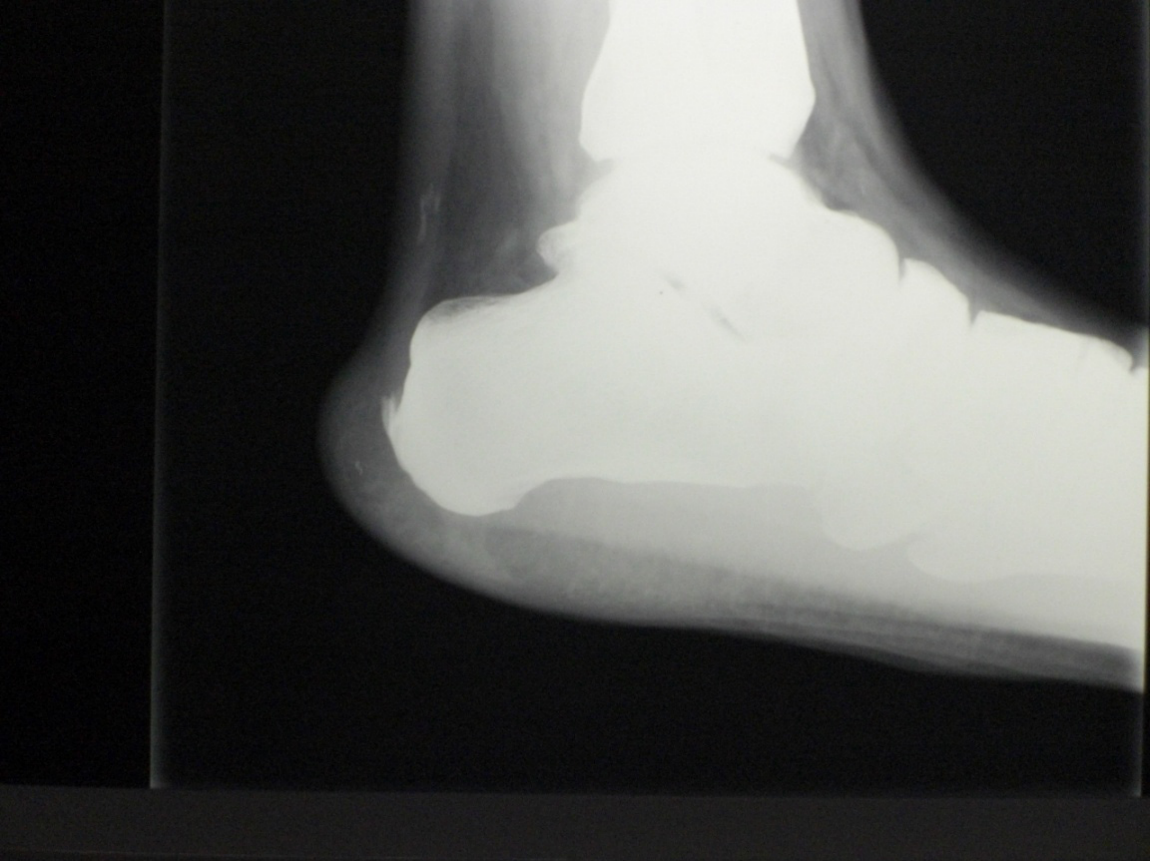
Insertional calcific tendinopathy (CT) of the Achilles tendon associated with CT of the main body of the tendon.
